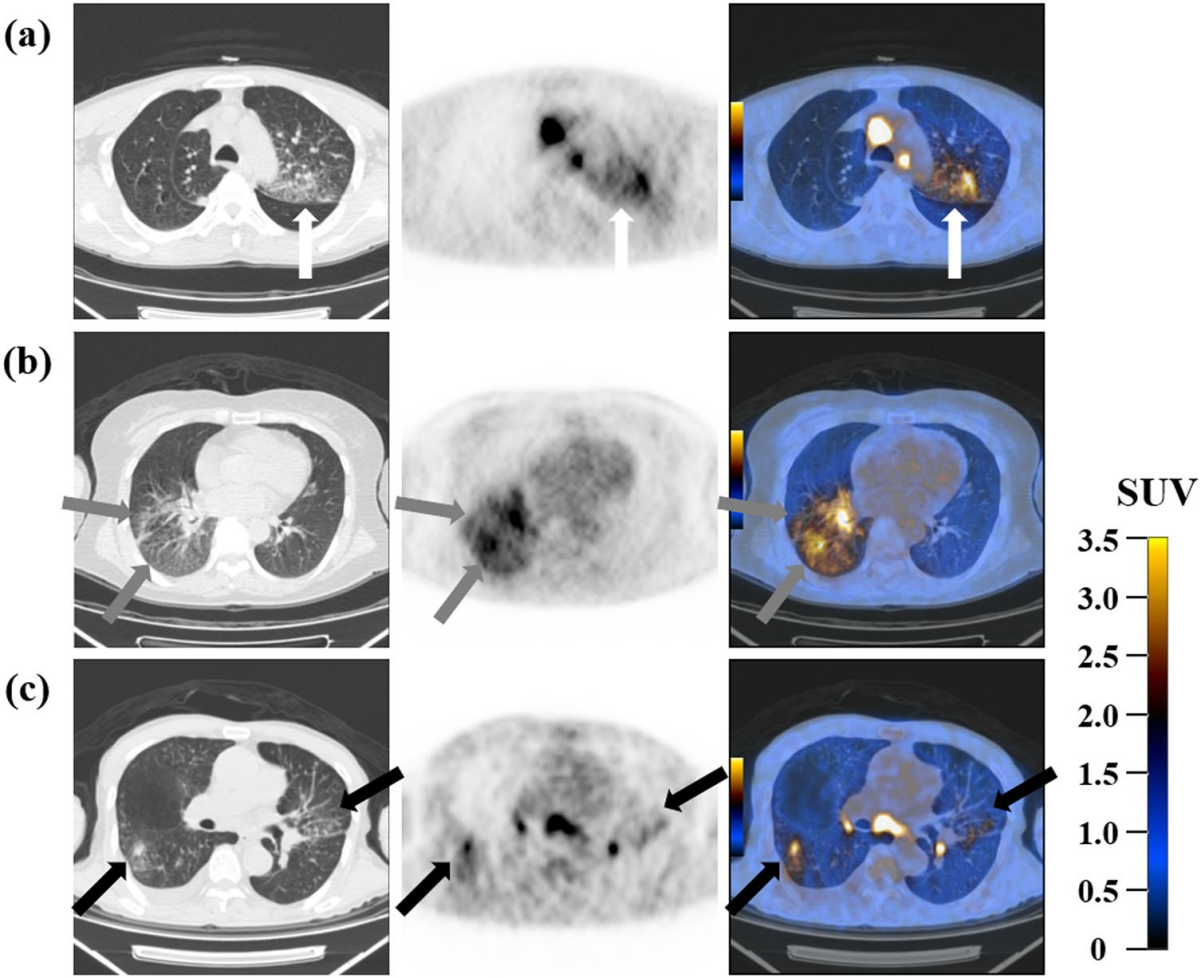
Representative FDG PET/CT images (CT, PET, and fusion images) of cPLC, classified as (a) cPLC1, (b) cPLC2, and (c) cPLC3. (a) The primary tumor was in left upper lobe and PLC was located only in the same lobe where the primary tumor is located (white arrows). PLC SUV_max_ in the left upper lobe and metabolic PLC burden were 3.27 and 3.27, respectively. (b) The primary tumor was in right lower lobe and PLC was confined to right lower lobe and right middle lobe (gray arrows). PLC SUV_max_ in right lower lobe and metabolic PLC burden were 3.06 and 6.12, respectively. (c) The primary tumor was in left upper lobe and PLC was distributed throughout both lungs (black arrows). PLC SUV_max_ in left lower lobe and metabolic PLC burden were 3.77 and 18.85, respectively. *FDG* fluorine-18-fluorodeoxyglucose, *PET* positron emission tomography, *CT* computed tomography, *PLC* pulmonary lymphangitic carcinomatosis, *SUV*_*max*_ maximum standardized uptake value.
